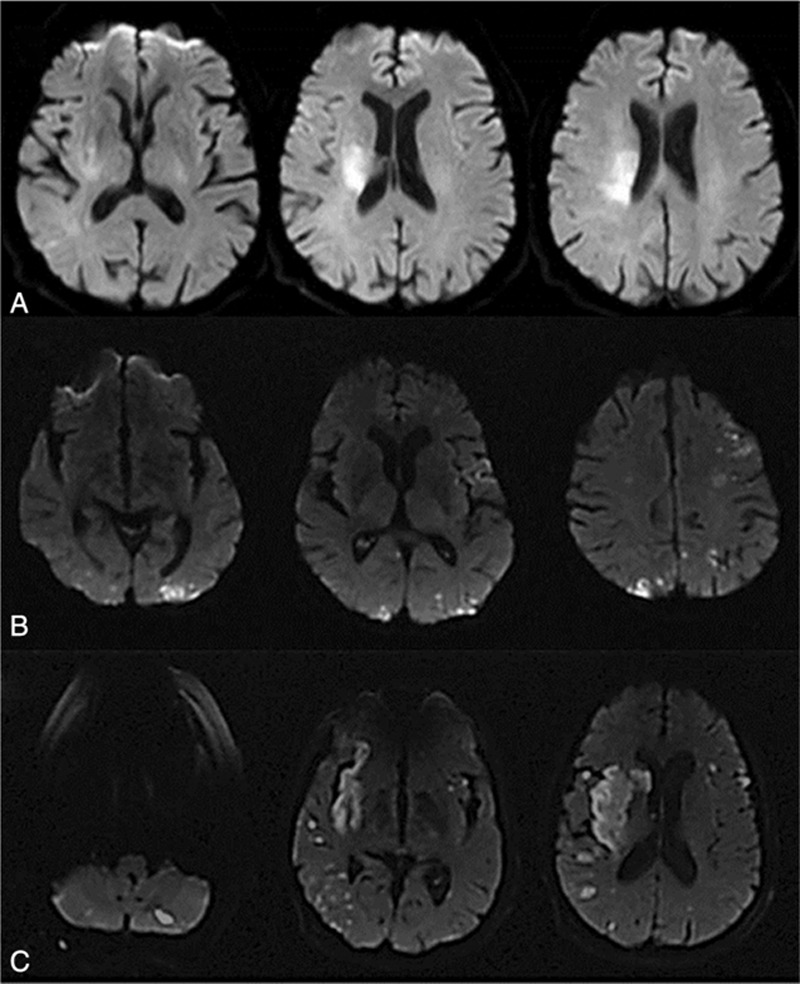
Brain diffusion-weighted magnetic resonance imaging showing radiological patterns of cerebral infarctions in patients with pancreatic cancer. A, Single infarctions (pattern 1); B, small disseminated infarctions (pattern 3); C, small and large disseminated infarctions (pattern 4). Patterns defined by Bang et al.^[12]^
